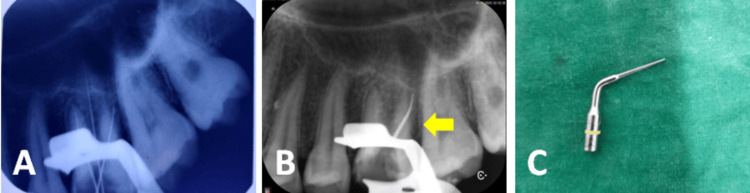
(A) Working length determination, (B) file separated in the distobuccal canal of 26, and (C) Satelec ET25 ultrasonic tip
